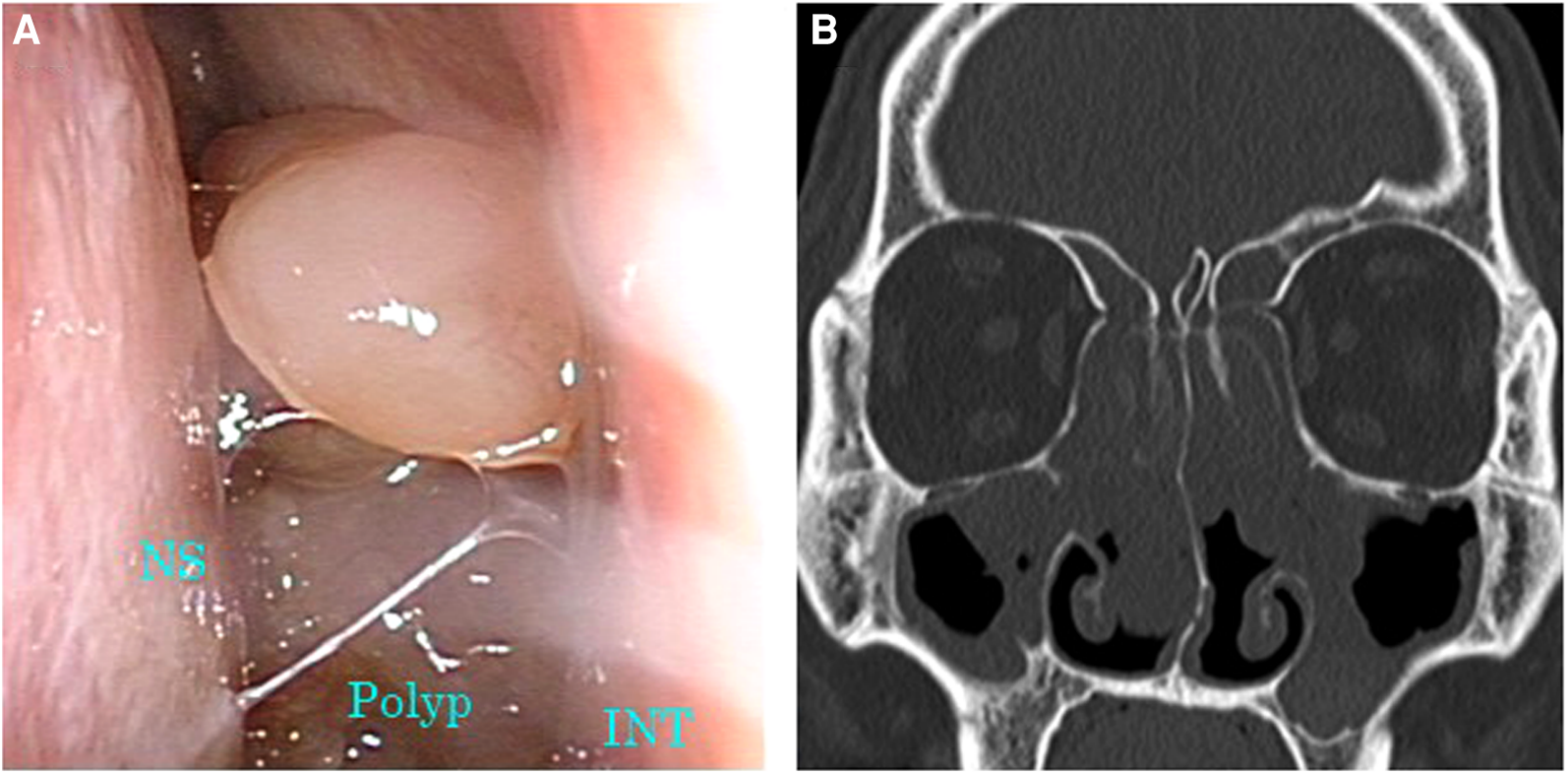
Images of nasal endoscopy and sinus CT before initiation of dupilumab therapy. (A) Nasal polyps occupying the common nasal meatus were observed. (B) Soft tissue density lesions were filling all sinuses on both sides. CT, computed tomography; INT, inferior nasal turbinate; NS, nasal septum.
